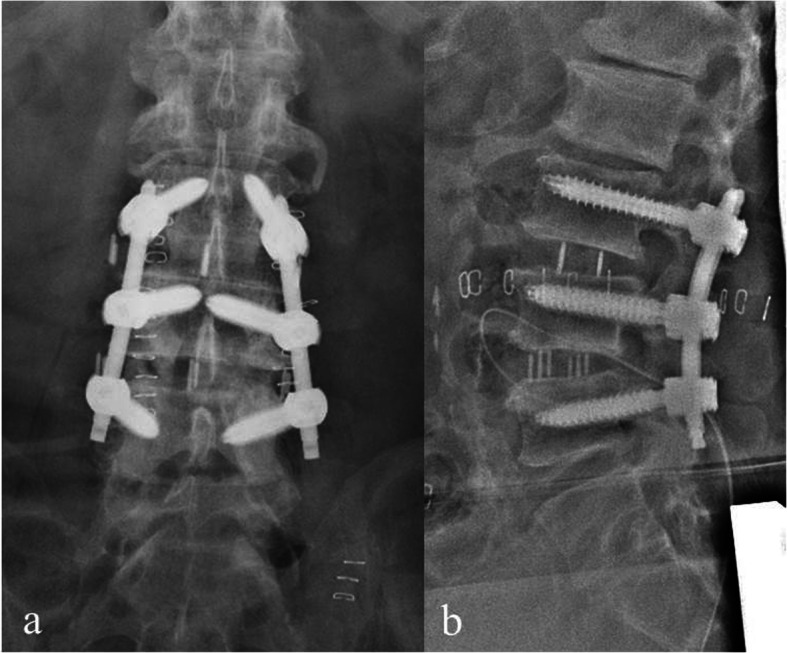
Postoperative radiographic imaging. Postoperative radiographic images a, b showing good alignment
